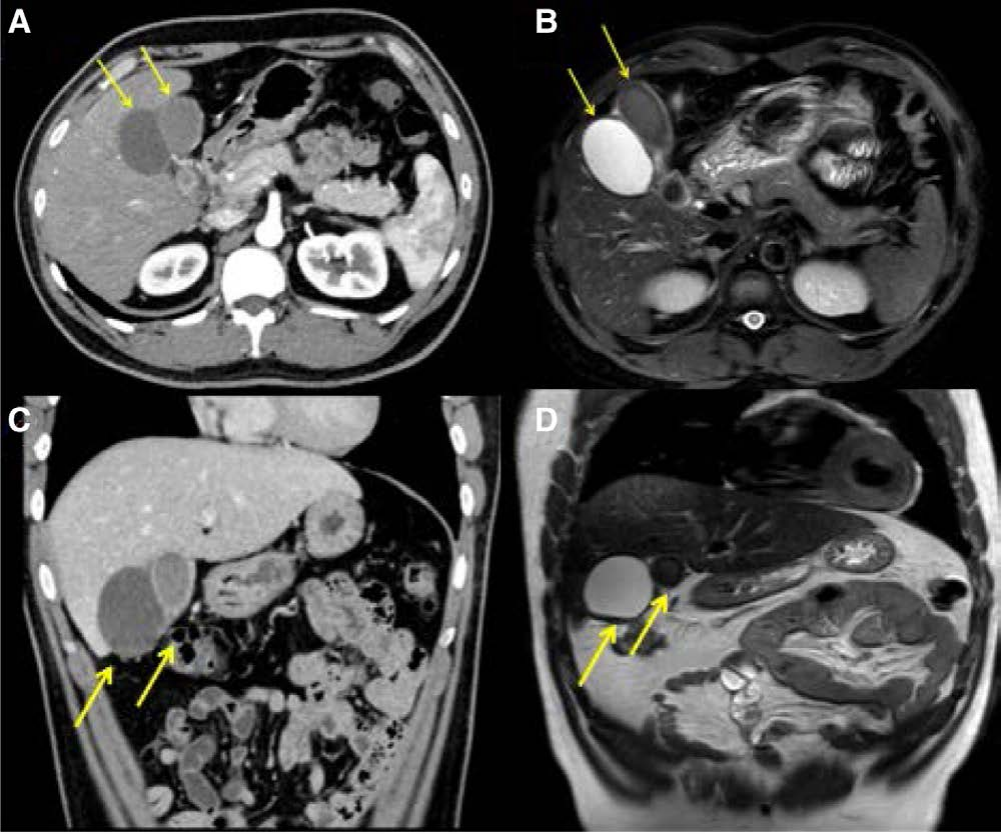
Computed tomography images and magnetic resonance imaging of the double gallbladders. (A) Abdominal CT revealed a 53 × 40 mm low density on the right gallbladder and a high-density shadow with an enhancing thick cystic wall on the left side of the gallbladder. (B) Abdominal MRI revealed a high possibility of a stone-packed double gallbladder but failed to visualize the cystic duct. (C) The coronal section of CT image of gallbladder (D) The coronal section of MRI image of gallbladder.
